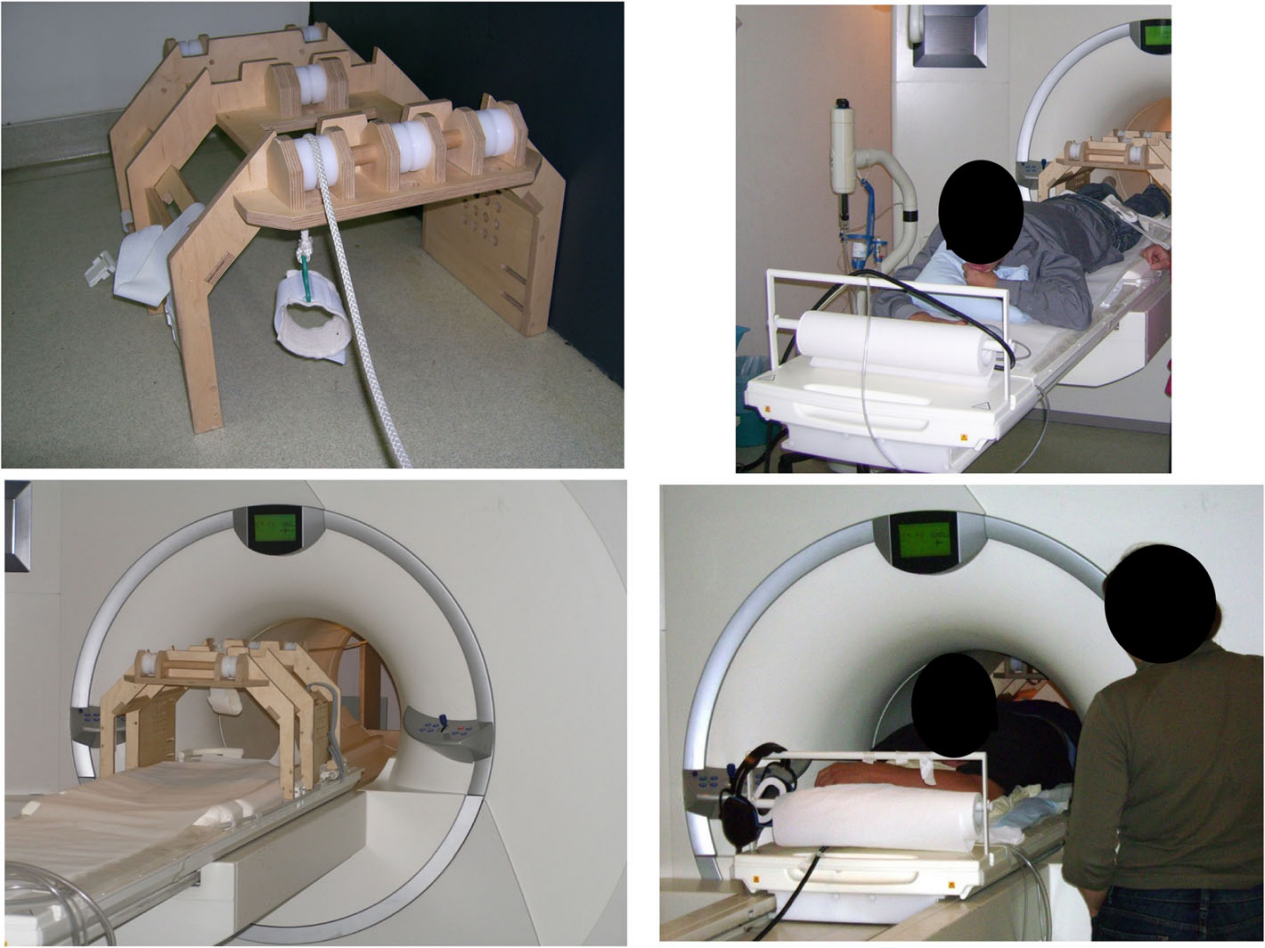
Self-built MRI compatible ergometer. Participants lay in prone position inside the MRI scanner. The ergometer was self-built and nonmagnetic (mainly built of wood). Moving of the work load was achieved via a pulley system. The left foot was secured to a padded foot loop. This loop was connected to a basket using a rope. Knee-extension led to an upward movement of the load. To ensure the correct placement of the thigh muscles on the magnetic coil, the thigh was secured to the coil using Velcro straps
